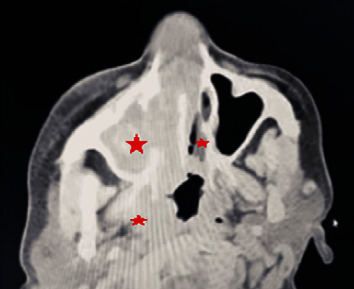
Computerized tomography (CT)-scan paranasal sinuses with sinuses with the area shown in the red star reveal nodular mucosal thickening in bilateral nasal cavities with widening and ^*∗*^showing destruction of right osteomeatal complex and pansinusitis.
